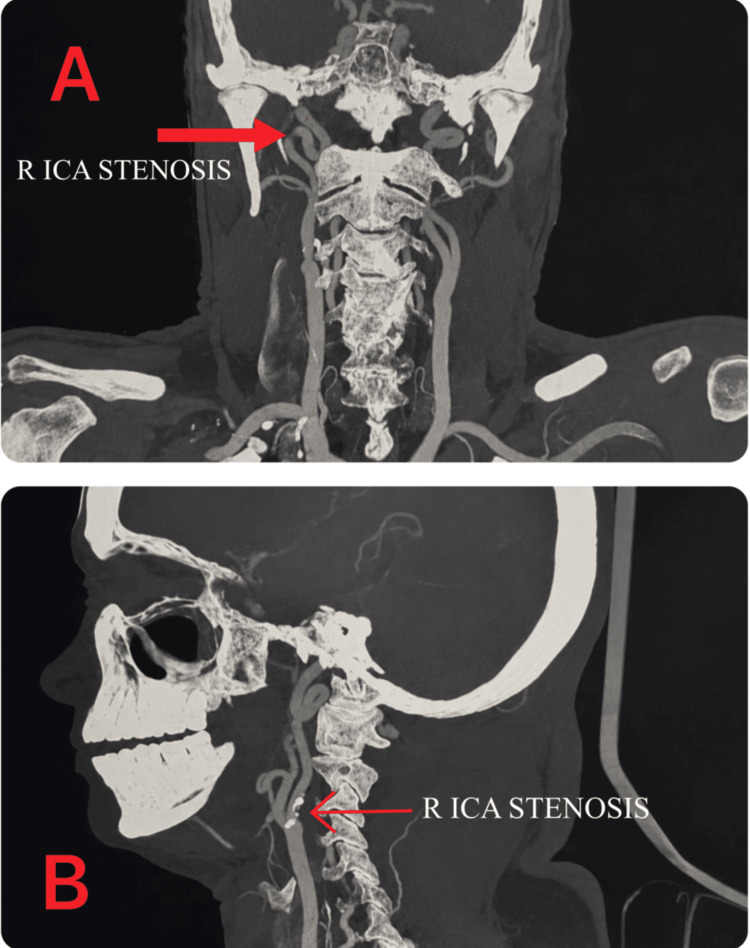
CT angiogram of neck vessels R ICA, right internal carotid artery
